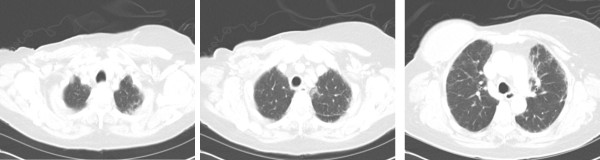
Computed tomography scan approximately three months later showing improvement of interstitial pneumonitis
